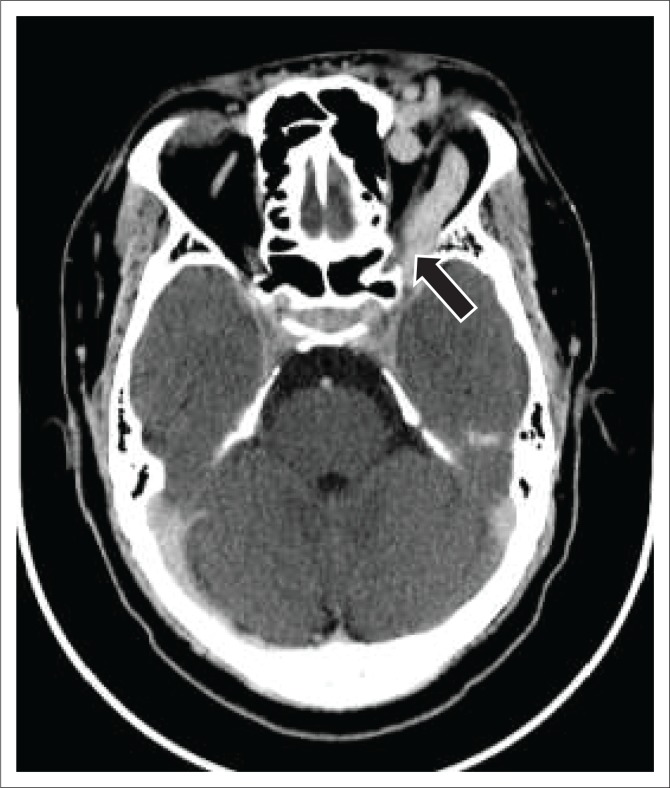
A 33-year-old male presented with a post-traumatic carotid cavernous fistula. Axial post-contrast computed tomography brain image demonstrates an enlarged tortuous left superior ophthalmic vein (arrow).
